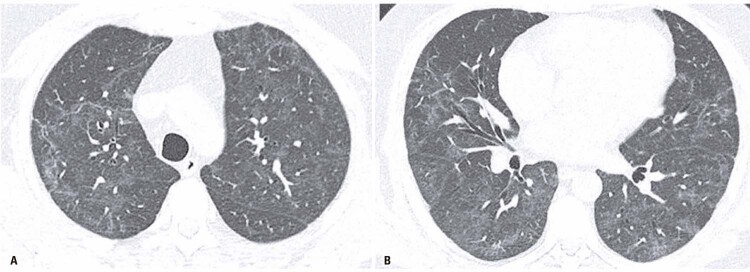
A and B) Non-fibrotic changes in a 49 years-old male patient, 85 days after symptoms onset, with normal diffusion capacity of carbon monoxide (106% of the predicted value). Chest computed tomography shows ground glass opacities with minimal linear abnormalities. There is no evidence of traction bronchiectasis, architectural distortion or honeycombing
